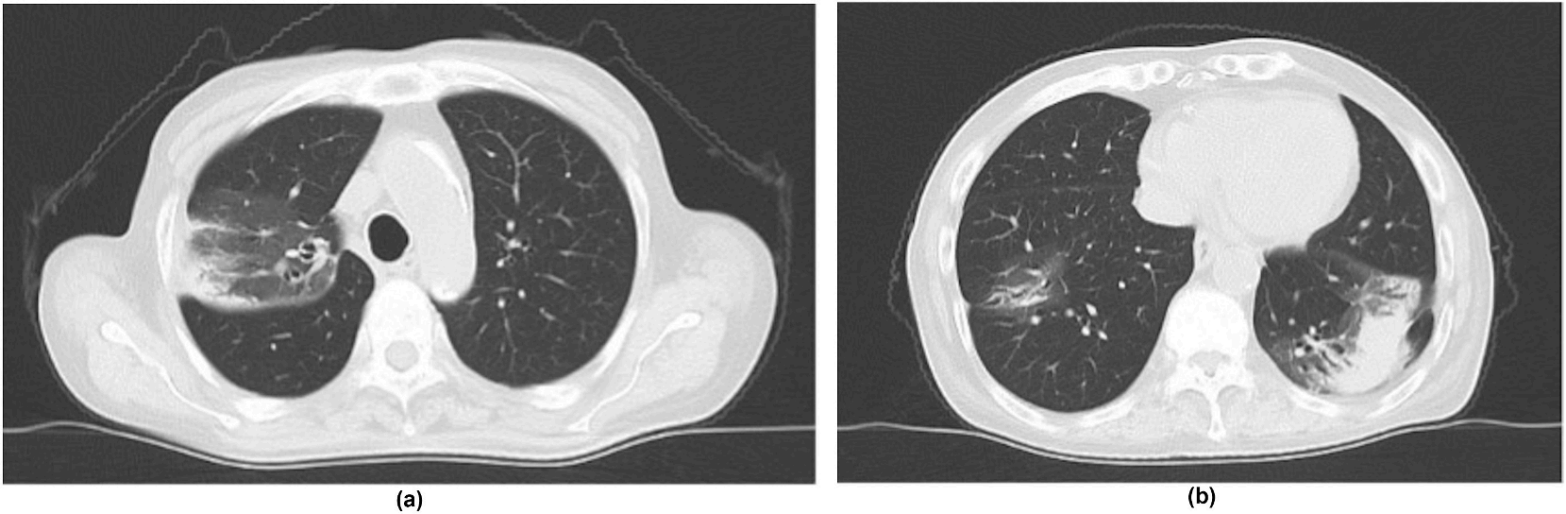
Chest CT scan 2 months after initiating corticosteroids and antifungal drugs. The infiltration shadows in both lung fields were reduced and pleural effusions disappeared.
